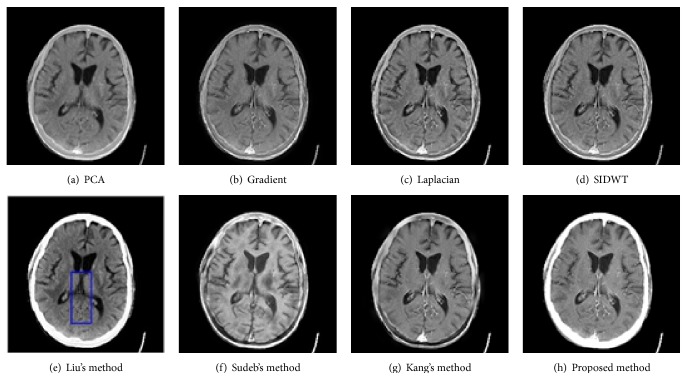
The fusion results of T1-MRI and GD-MRI.
